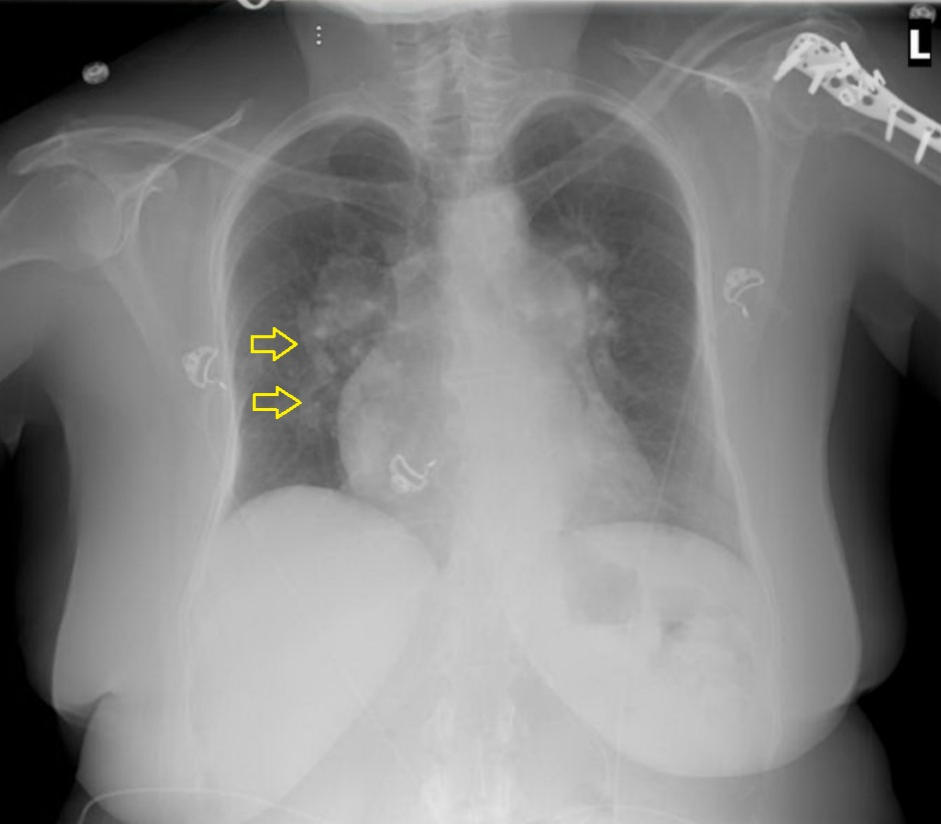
Chest X-ray demonstrating the Scimitar vein (marked by yellow arrow).
